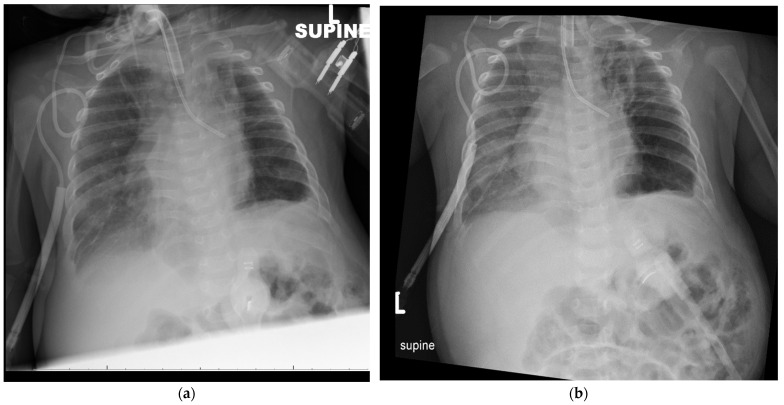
Chest radiographs after placement of bronchial blocker. Placement of a bronchial blocker in the left bronchus, resulting in better expansion of the right lung 6 h (a) and 24 h (b) after placement of blocker.
